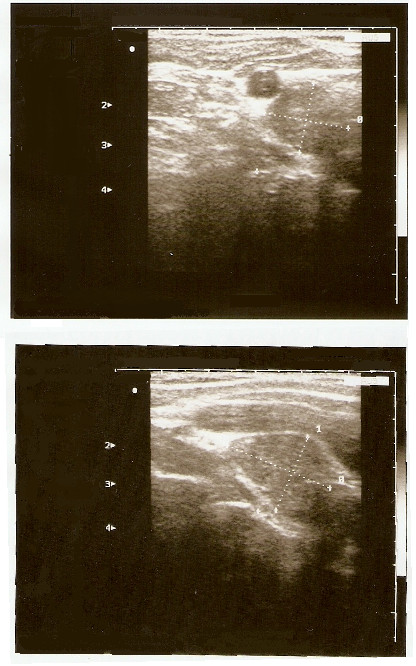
Neck ultrasonography images obtained about 21 months previously, demonstrating a solid cervical mass of 14 × 16 × 27 mm, with intra-lesional vascularization, under the right thyroid lobe. After fine-needle aspiration biopsy (FNAB), parathyroid hormone (PTH) evaluation on fine-needle washing was 550 pg/mL; this value was higher than our institutional standard cut-off value of 132 pg/mL [30].
